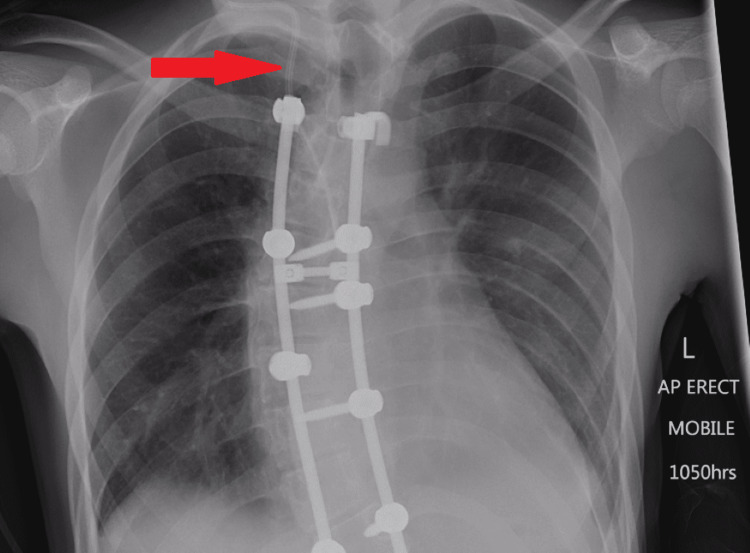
Chest X-ray performed for septic screen. The internal jugular vein central venous catheter is visible as indicated by the arrow.
